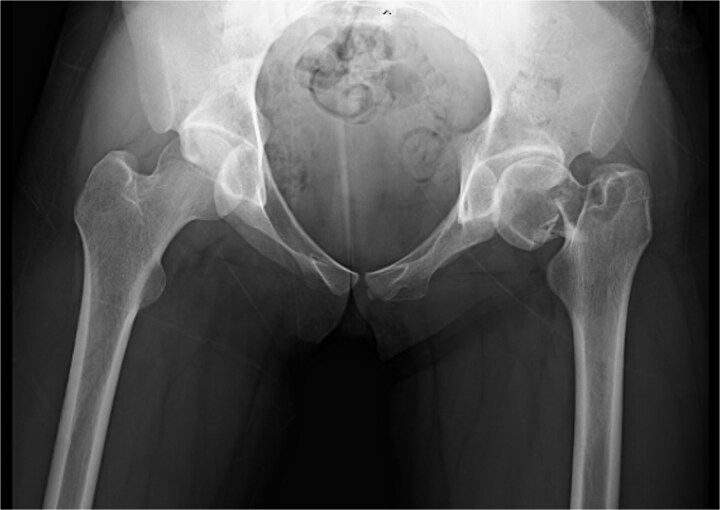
Plain radiograph at the time of injury revealed a Garden IV femoral neck fracture.
